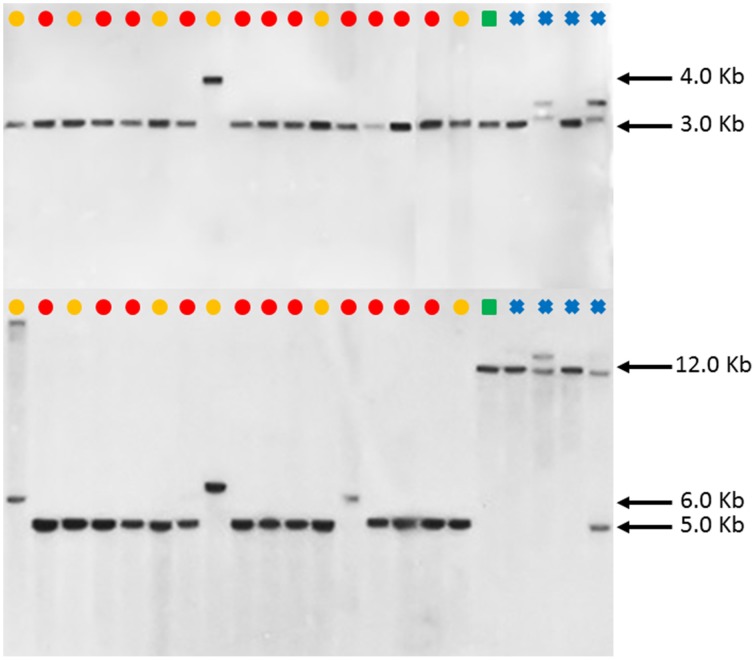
Southern blot *FAD2-2* digestion radiographs (top using enzyme *Bgl*II, bottom using enzyme *Hin*dIII). Samples are in order according to their listing in Table 1 starting with PI 209295 and ending with PI 426488.
